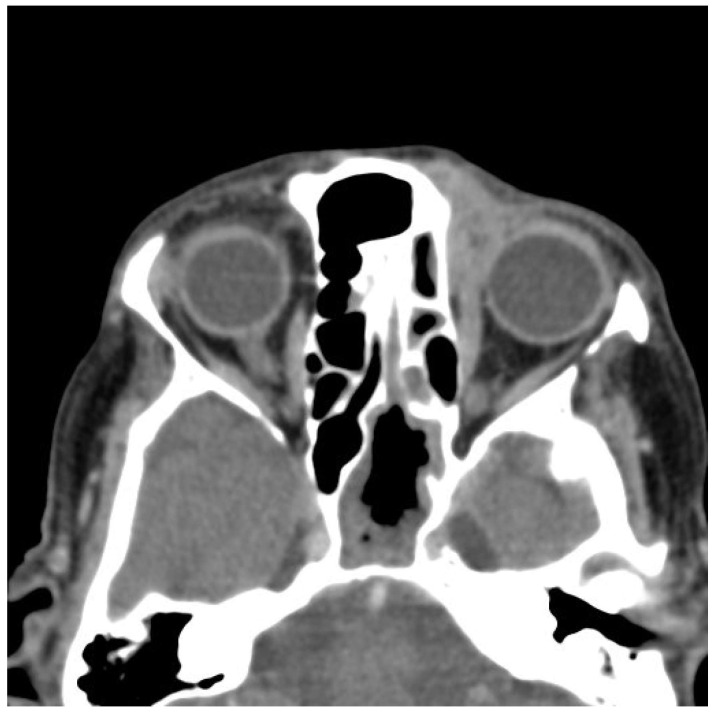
Local recurrence detected on follow-up CT scan.
